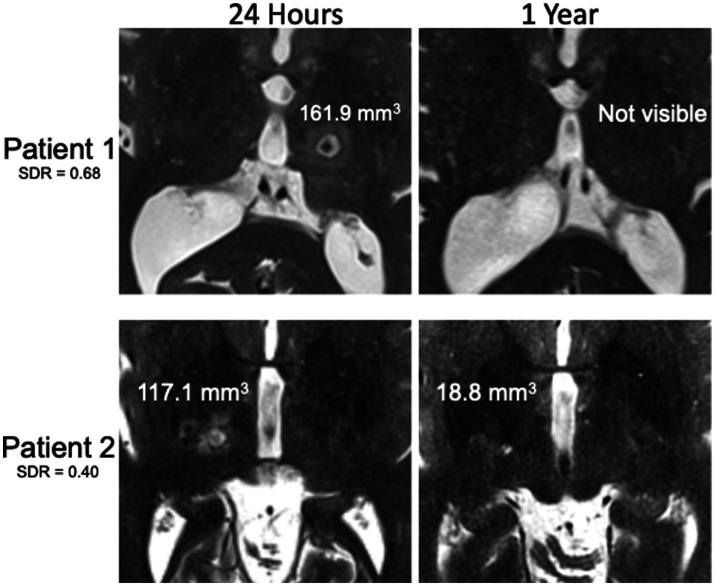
Examples of T2-weighted MR images 24 h and 1 year after MRgFUS for essential tremor. Patient with high SDR without visible lesion at 1 year despite large lesion at 24 h (top) compared to patient with low SDR with visible lesion at 1 year despite smaller lesion at 24 h (bottom). Lesion volumes displayed on the images.
